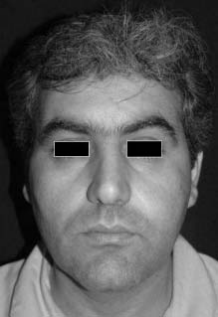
CT scan of the upper jaw showed an osteolytic mass with extensions to surrounding soft tissues. The margins were ill-defined (Figure 2).
